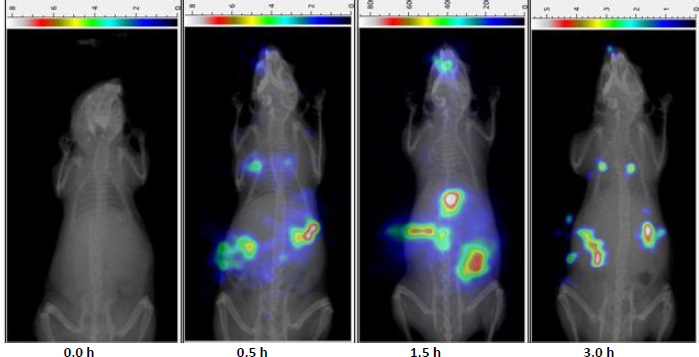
Bimodal SPECT-CT Images indicating brain distribution of MCNE following intranasal administration (0-30 h)
